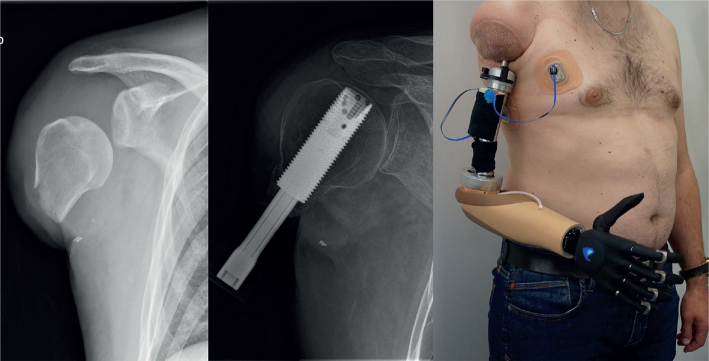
AP X-ray of the remaining humerus bone before targeted muscle reinnervation and osseointegration surgery (left), AP X-ray 16 weeks after stage 2 surgery (middle) and after prosthetic fitting with adhesive electrodes in patient 1 (right).
